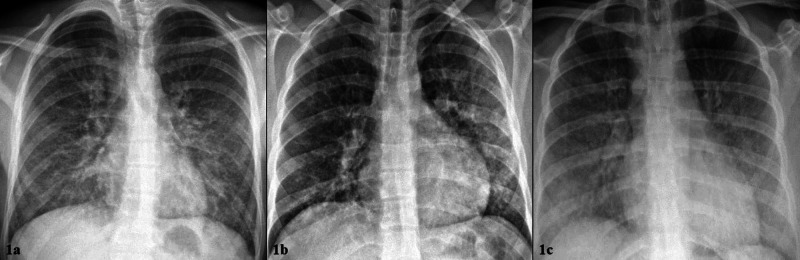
a-c: Initial chest X-ray at presentation. From left to right: Patient 1, Patient 2, Patient 3. 1a “Extensive bilateral ground glass and interstitial lung opacities.” 1b “Extensive bilateral patchy opacities, greater in the left lung.” 1c “Diffuse, bilateral, ground glass lung opacities with hazy appearance, vascular congestion.”
